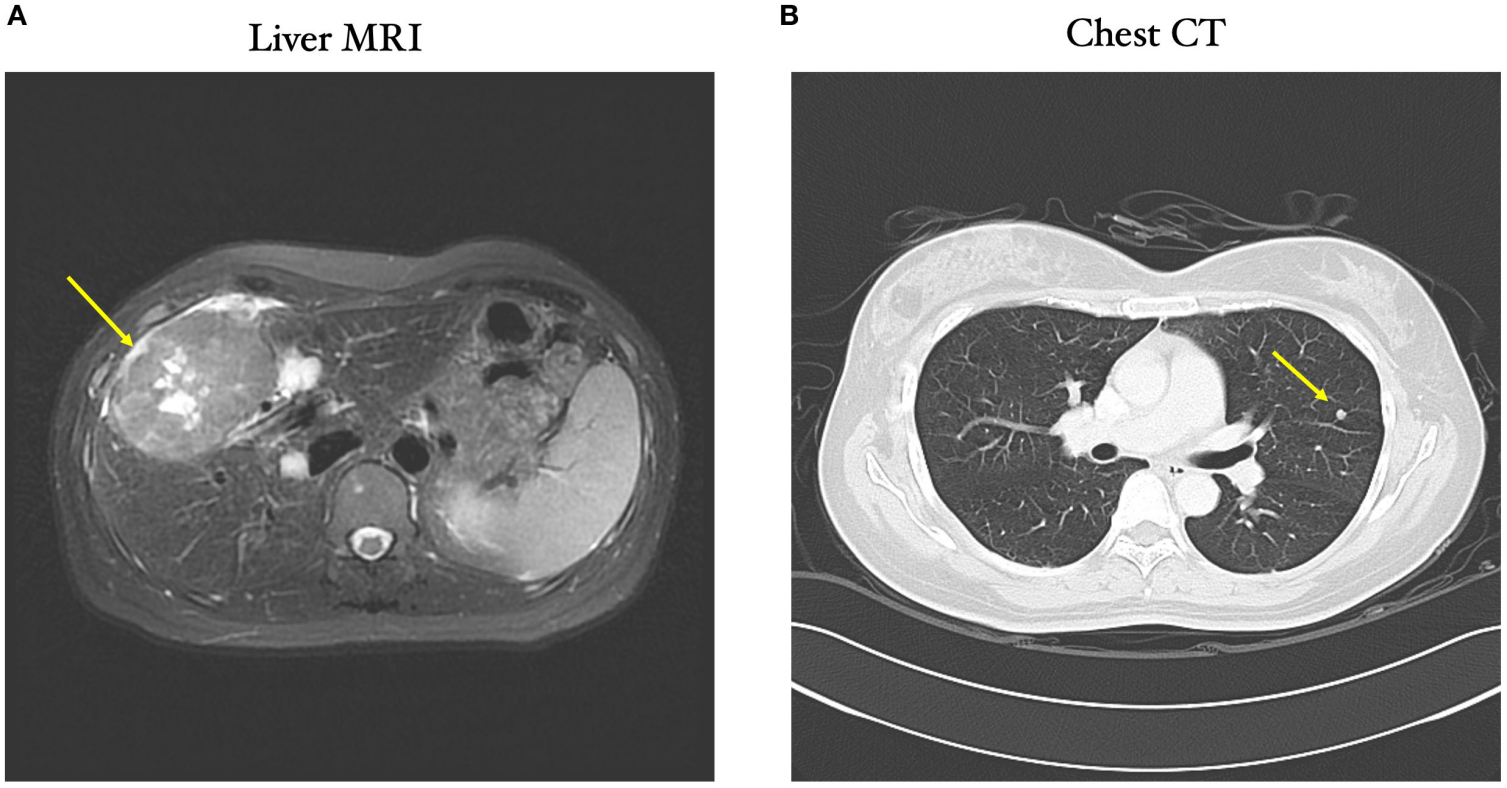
Liver MRI and CT scans at the time of the initial patient diagnosis. (A) MRI scan showing lobulated soft tissue mass predominantly at right lobe of liver, with mild high signal intensity on T2 weighted image (T2WI) (yellow arrow) and (B) CT scan showing nodular shadow at right upper lobe of lung (yellow arrow). A T2WI is a standard MRI sequence used for evaluating focal liver lesions. HCC typically shows higher signal intensity on T2WI compared to normal liver parenchyma.
